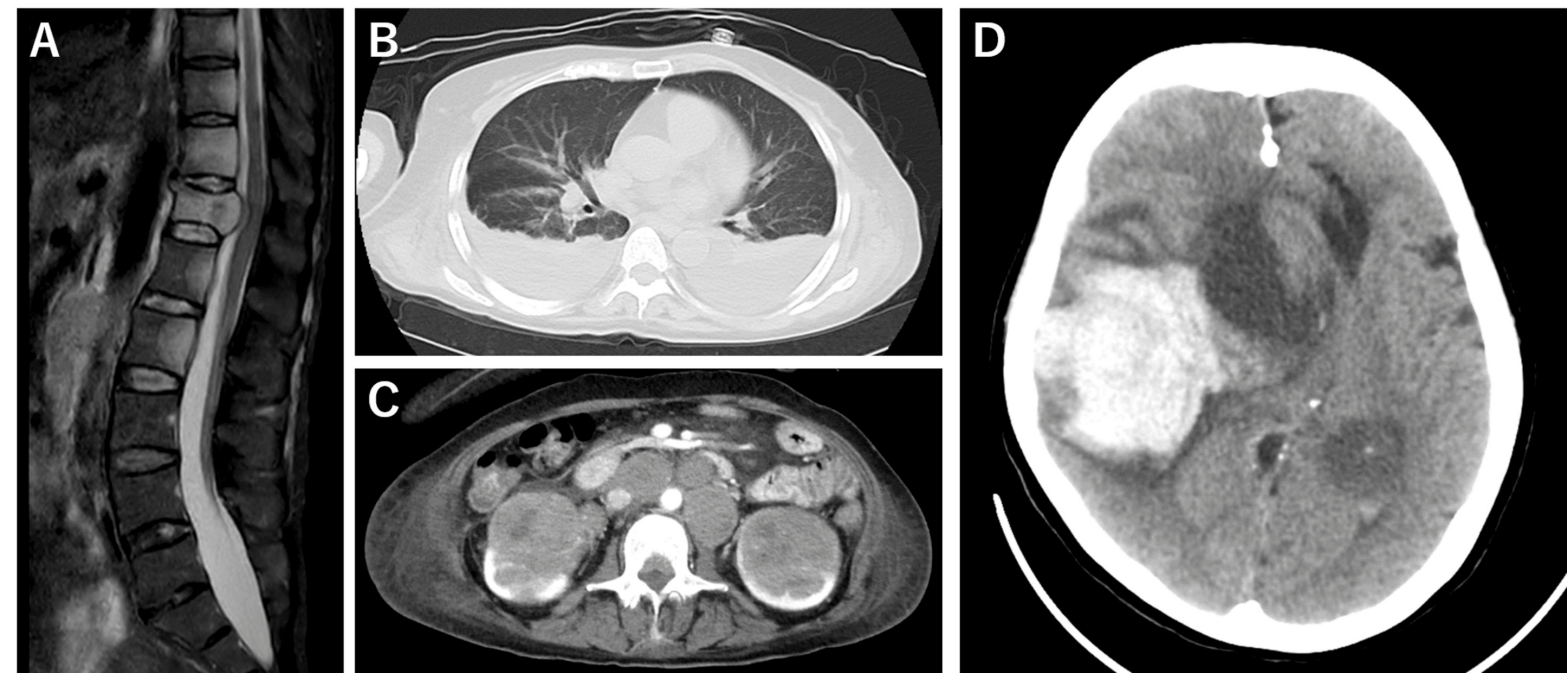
Imaging tests during the third hospitalization (days 212-221) (A) Spine magnetic resonance imaging (MRI). (B) Pulmonary computed tomography (CT). (C) Contrast-enhanced abdominal CT. (D) Brain CT performed after sudden loss of consciousness.
